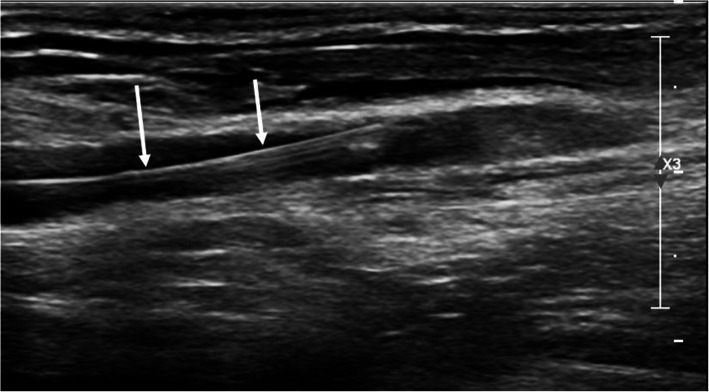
Longitudinal ultrasound picture of 19G puncture needle and guidewire (white arrows), which was inserted under live ultrasound guidance
